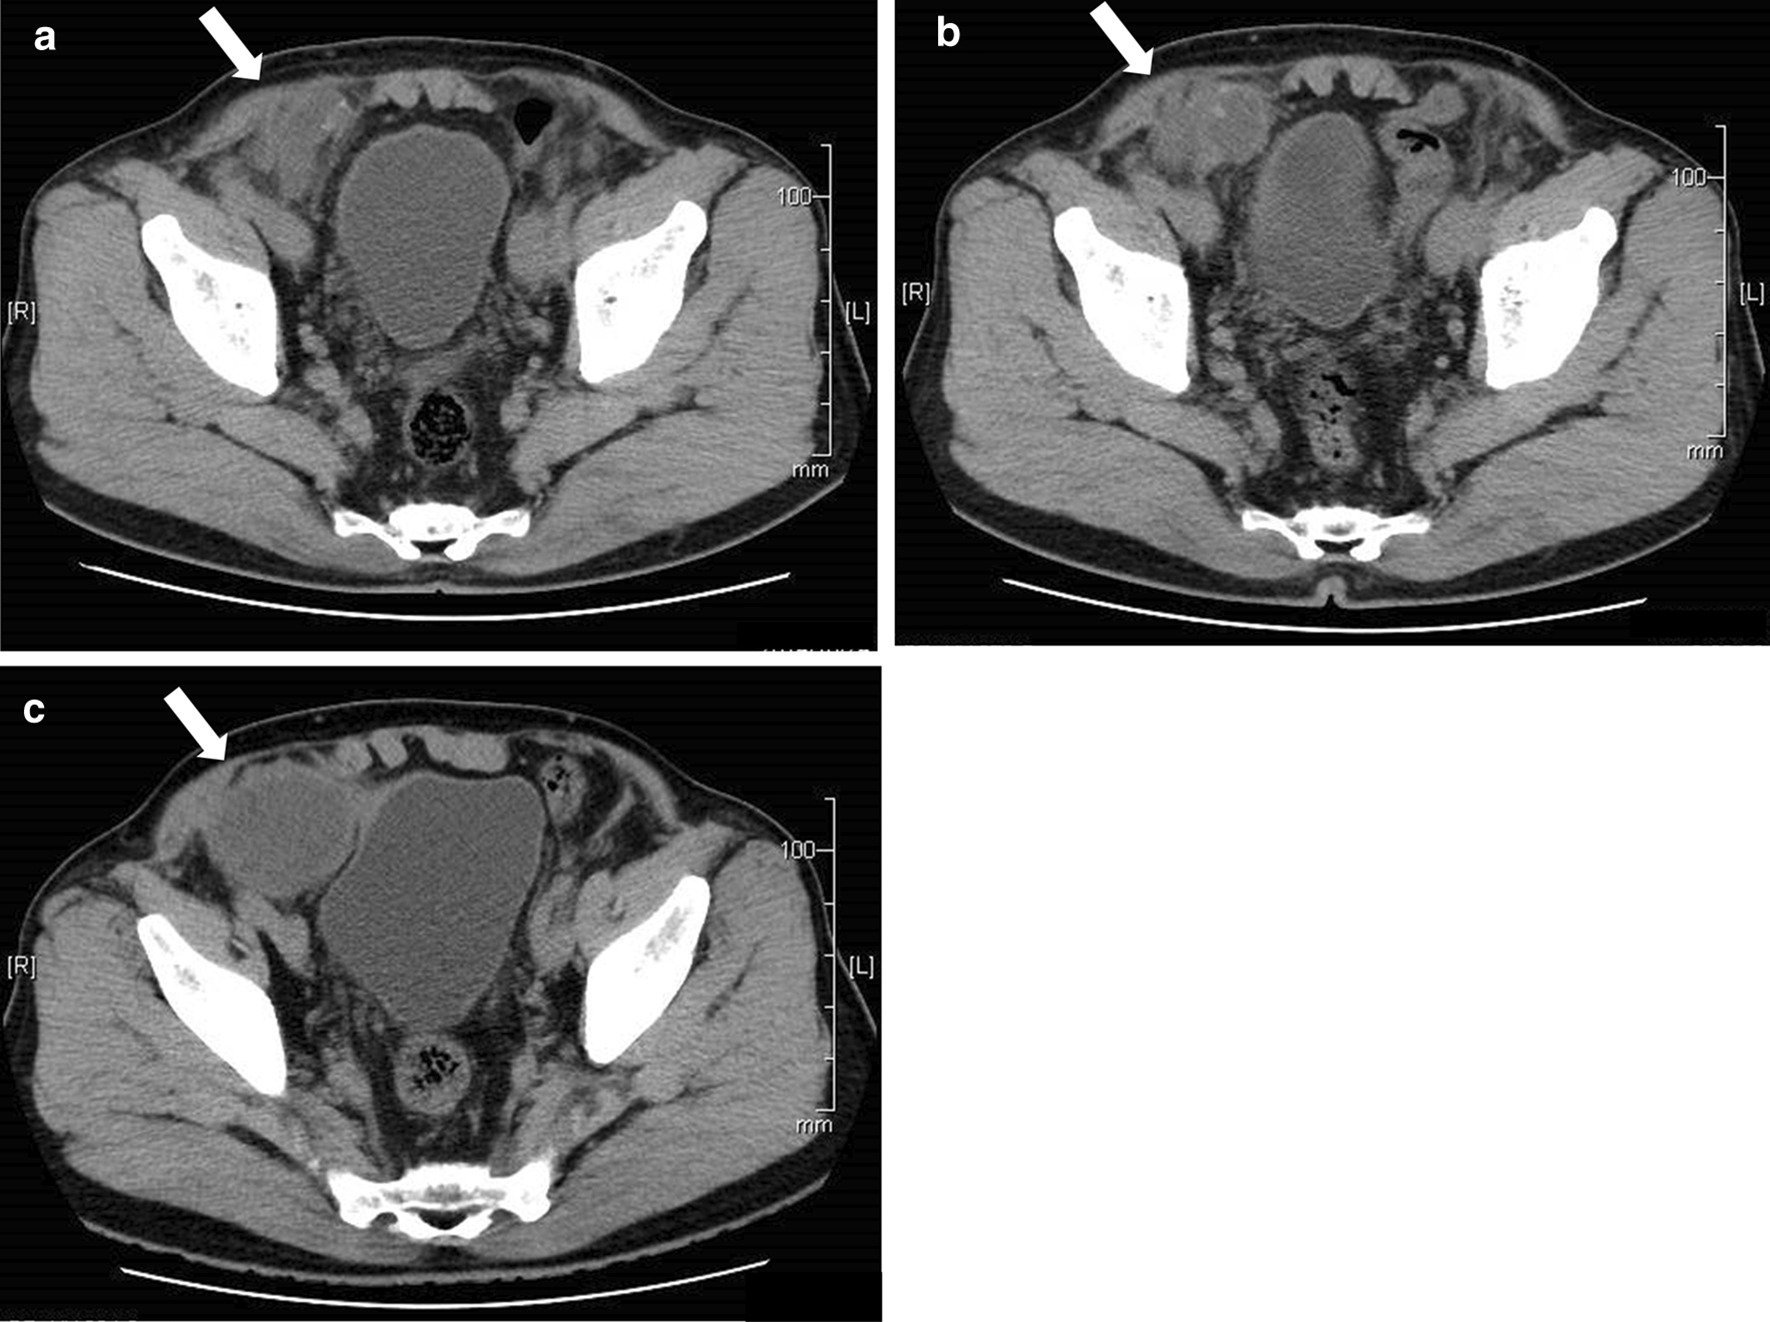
Computed tomography shows fluid collection in the right inguinal lesion (arrow, fluid collection) a 1 month after surgery, b 3 months after surgery c 10 months after surgery
